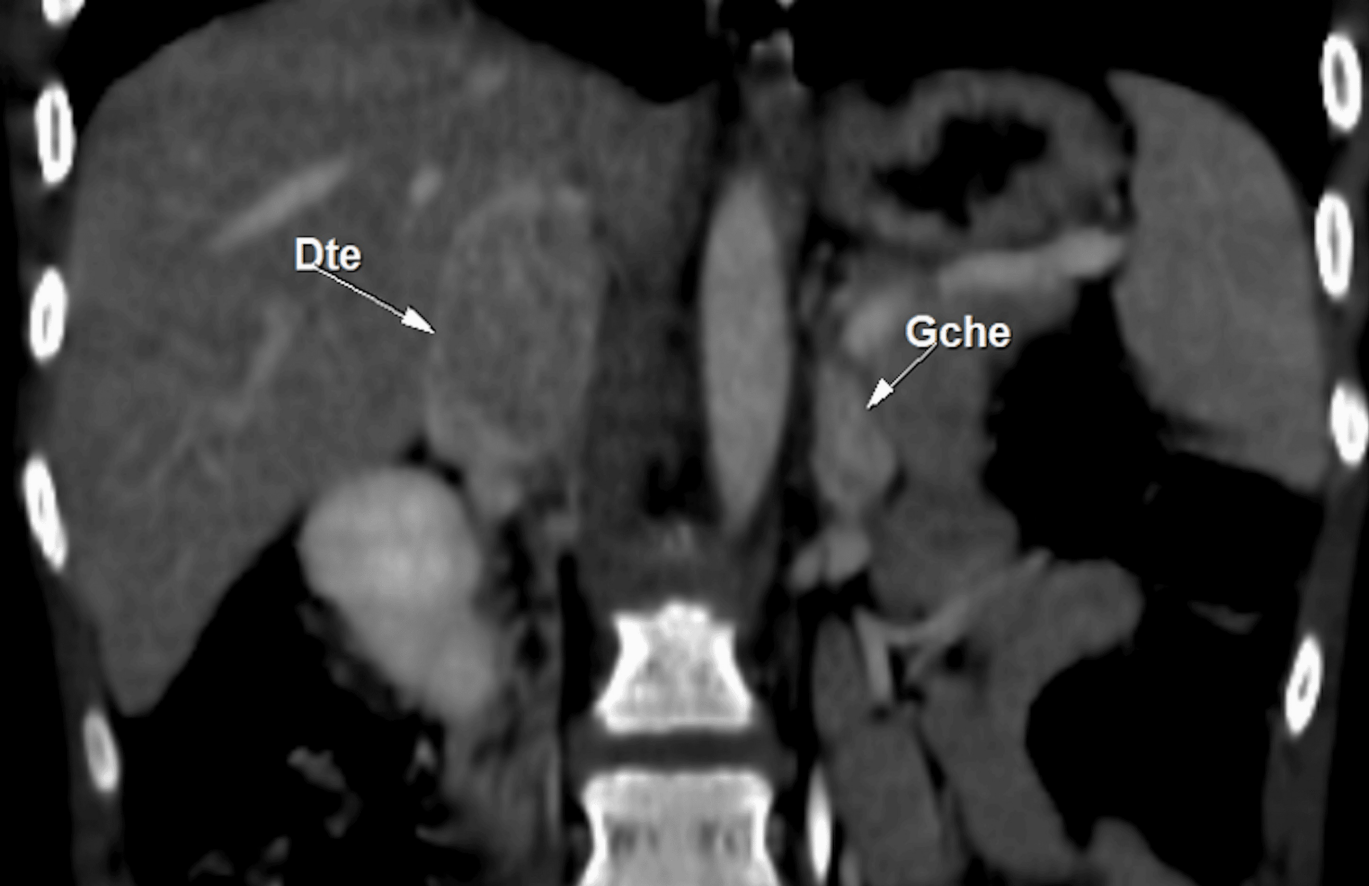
Coronal CT scan showing bilateral adrenal masses right: 3.5 × 2.5 cm; left: 13 × 12 mm CT: computed tomography
